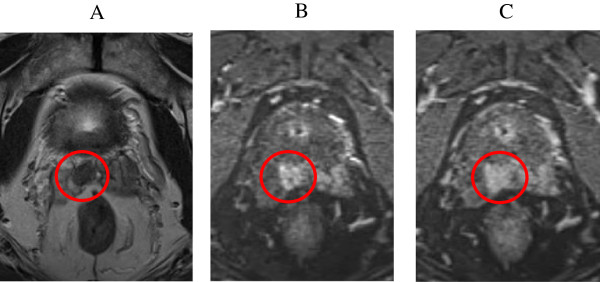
MRI images of seminal vesicle recurrence. (A) T2-weighted image demonstrated a low-intensity area in the bottom of seminal vesicle. (B, C) Dynamic MRI also demonstrated a recurrence of seminal vesicle because of an intensive enhancement in early phase and a washed-out in late phase.
